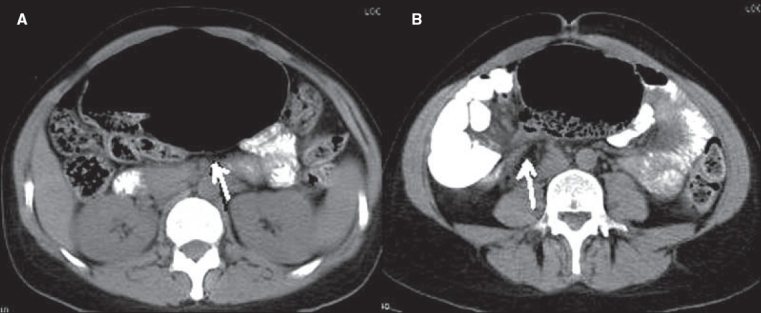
Cecal volvulus. Plain CT study shows a dilated cecum (arrow in A) with an air-fluid level reaching up to the left upper abdomen, demonstrating a beak sign (arrow in B). The appendix is not definitely visualized
